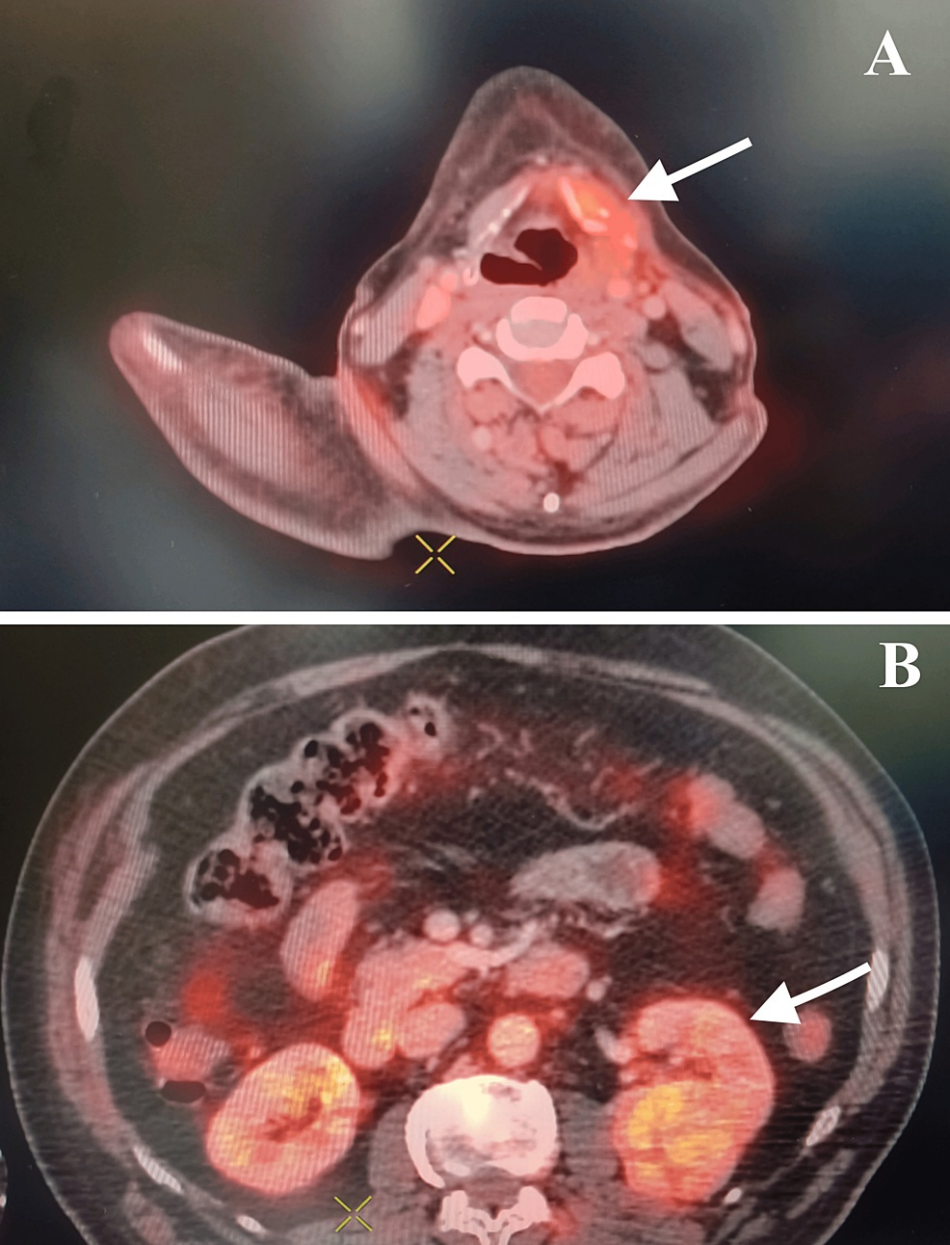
(A) An 18F-FDG PET-CT scan showing thickening with FDG uptake in thyroid cartilage and (B) in the left kidney (white arrows). FDG PET-CT, fluorodeoxyglucose positron emission tomography-computed tomography
